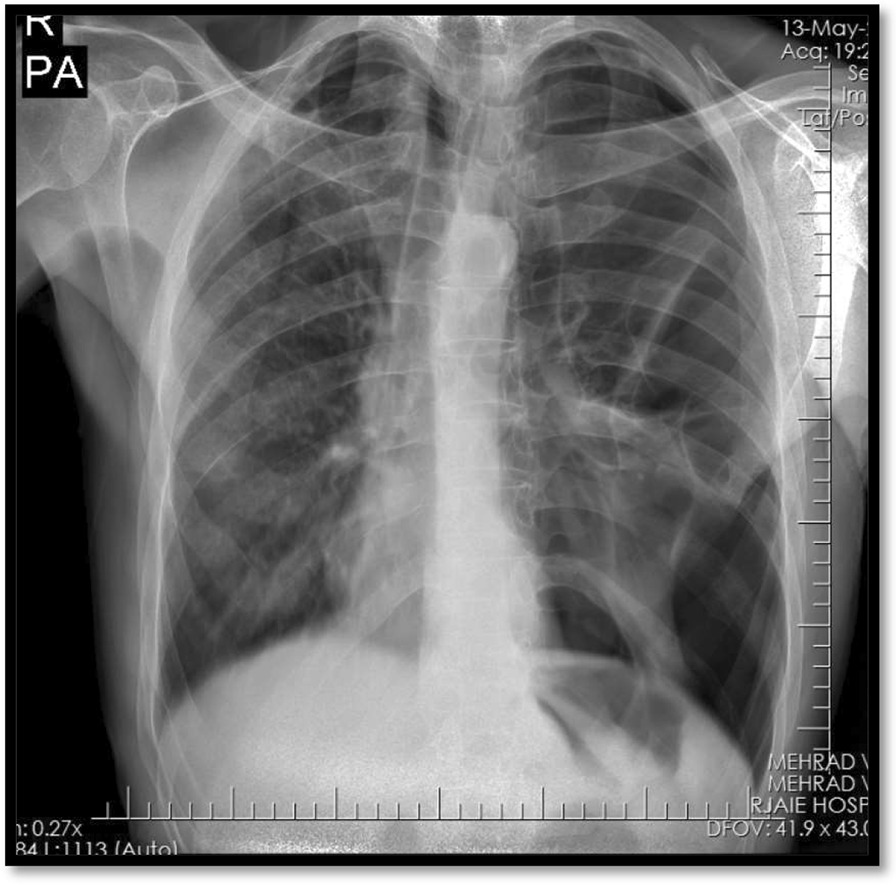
Chest radiography (2nd admission), left sided recurrent spontaneous pneumothorax
